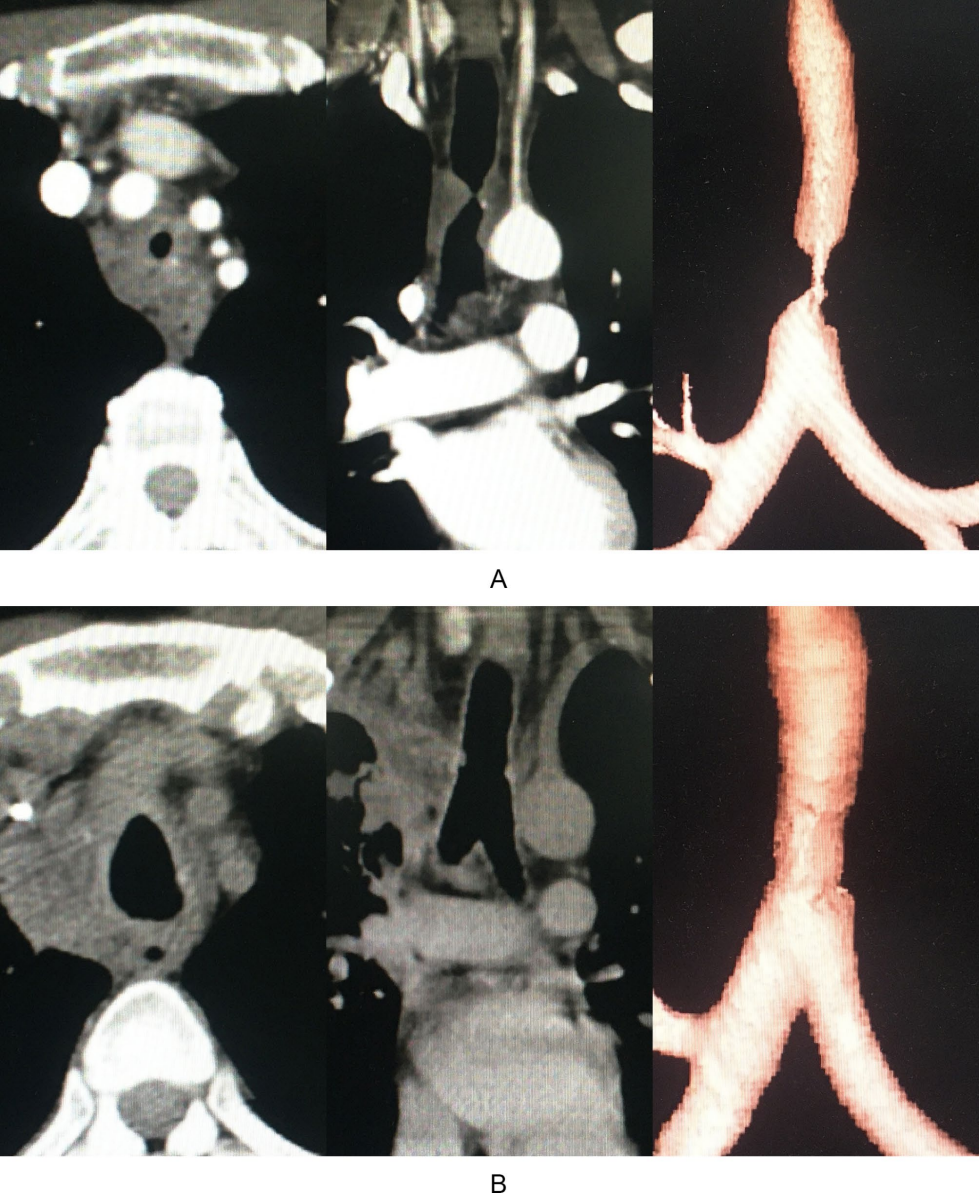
(A) CT scan showed the severe TS in case #13 prior to operation; (B) CT scan showed the reconstructed trachea
